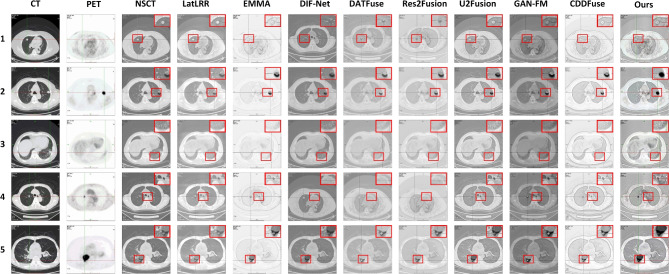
The comparison experiments fusion results of CT lung window images and PET images. Method 1: NSCT; Method 2: LatLRR; Method 3: EMMA; Method 4: DIF-Net; Method 5: DATFuse; Method 6: Res2Fusion; Method 7: U2Fusion; Method 8: GAN-FM; Method 9: CDDFuse; Method 10: Ours.
